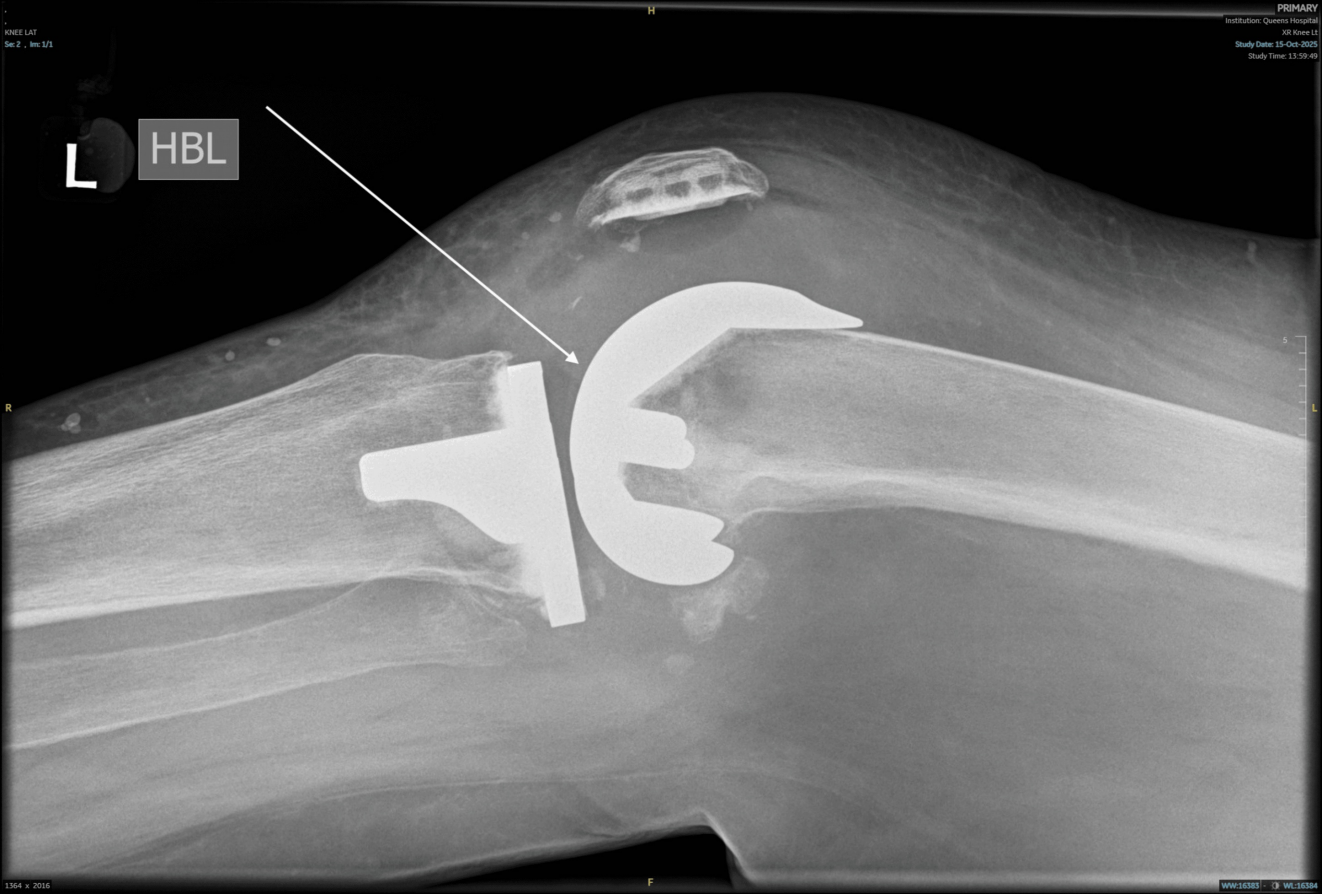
Lateral radiograph of the left knee obtained prior to aspiration, demonstrating the total knee replacement prosthesis in situ. The arrow indicates the total knee replacement prosthesis in situ
